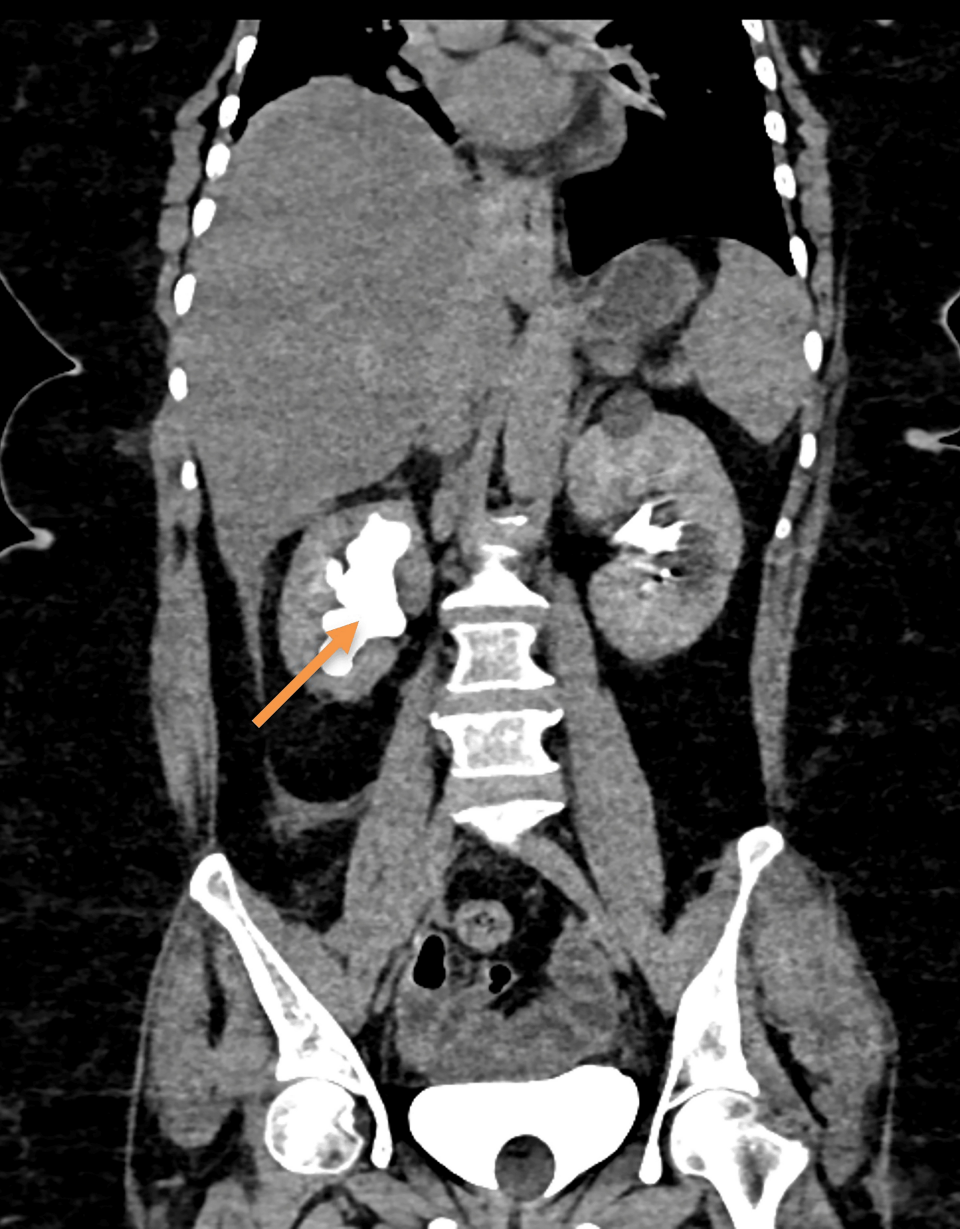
CECT abdomen (coronal section) of the patient. The arrow shows a dilated right pelvicalyceal system (Grade 3 hydronephrosis) with a sudden narrowing of the right proximal ureter. CECT: Contrast-enhanced computed tomography
